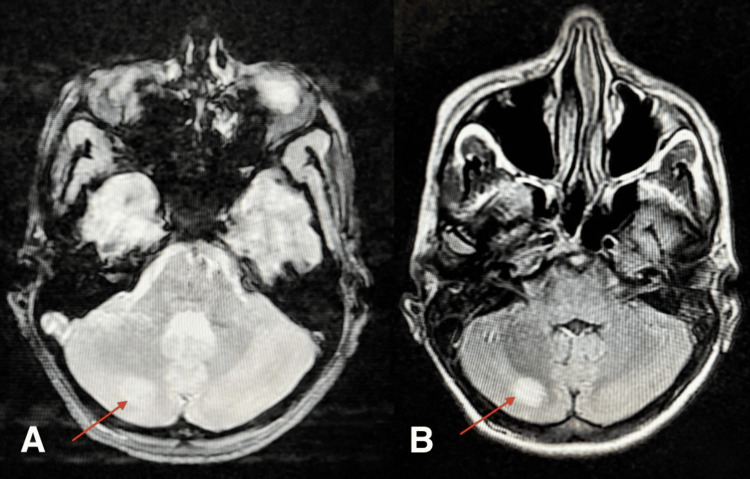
Axial brain MRI sequence of the patient, showing right cerebellar ischemic lesion. (A) T2-FLAIR sequence showing the same cerebellar lesion (red arrow). (B) T2-weighted sequence showing an acute ischemic lesion in the right cerebellar hemisphere (red arrow). MRI: Magnetic Resonance Imaging; FLAIR: Fluid-Attenuated Inversion Recovery
